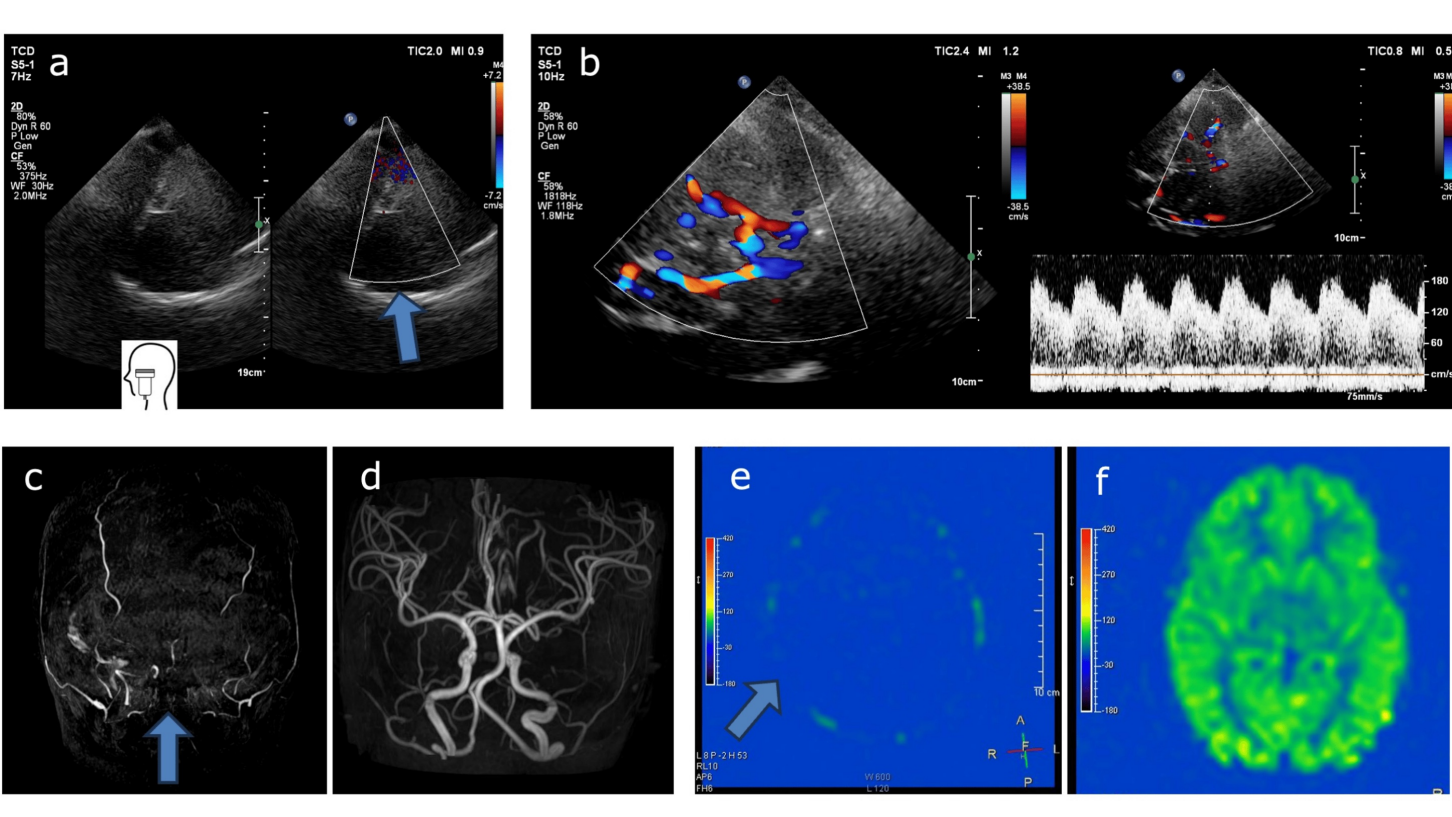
Comparison with normal findings in Transcranial Doppler (TCD) examination and MRI a. Even on reducing the color Doppler velocity to the minimum value, no blood flow was detected bilaterally. b. Representative normal TCD in another case. c. No blood flow was observed on magnetic resonance angiography (MRA). d. Representative normal MRA in another case. e. No blood flow was detected on arterial spin labeling (ASL). f. Representative normal ASL in another case.
